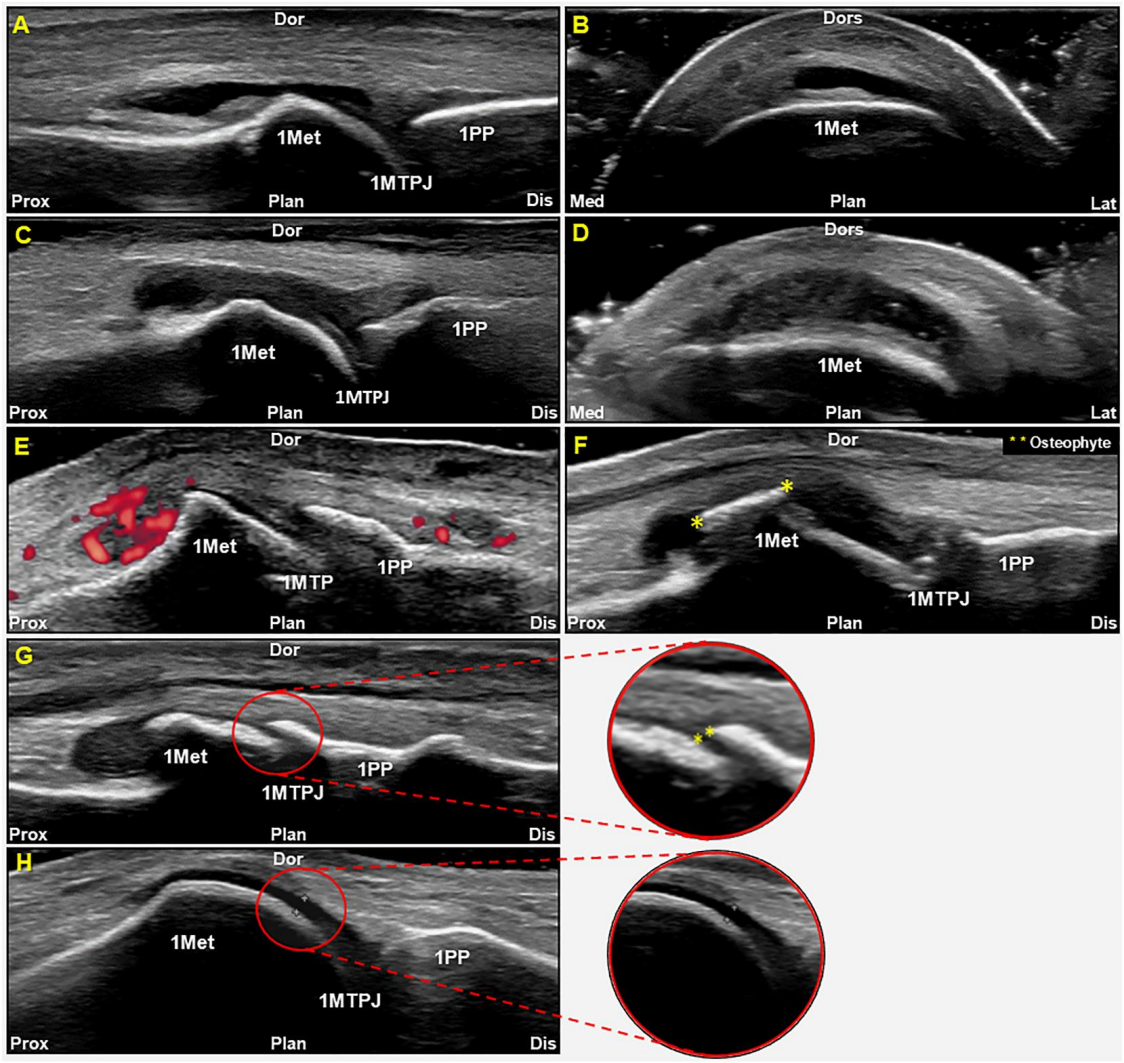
Ultrasound imaging first MTPJ osteoarthritis features that were examined. (A) Joint effusion in longitudinal; (B) joint effusion in transverse; (C) synovial hypertrophy in longitudinal; (D) synovial hypertrophy in transverse; (E) synovitis; (F) osteophyte; (G) joint space narrowing; (H) cartilage thickness. 1Met, first metatarsal; 1MTPJ, first metatarsophalangeal joint; 1PP, first proximal phalanx; Dis, distal; Dor, dorsal; Lat, lateral; Med, medial; Plan, plantar; Prox, proximal.
